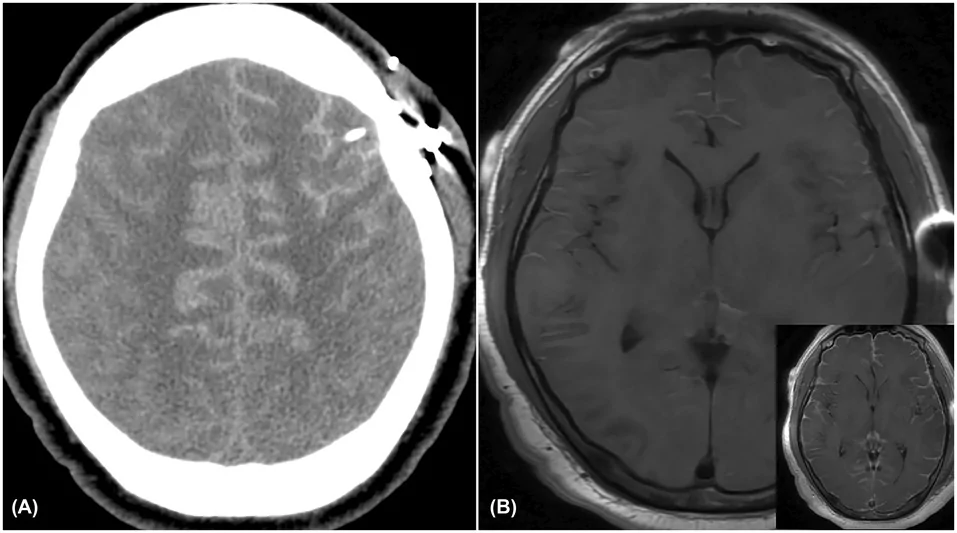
Imaging features. (A) Non‐contrast CT reveals increased density of the sulci primarily in the frontal lobes. (B) MRI axial T1 pre‐contrast shows increased intrinsic T1 signal along the sulci. Inset (lower right): axial T1‐weighted post‐contrast MRI reveals prominent, superimposed diffuse leptomeningeal enhancement.
